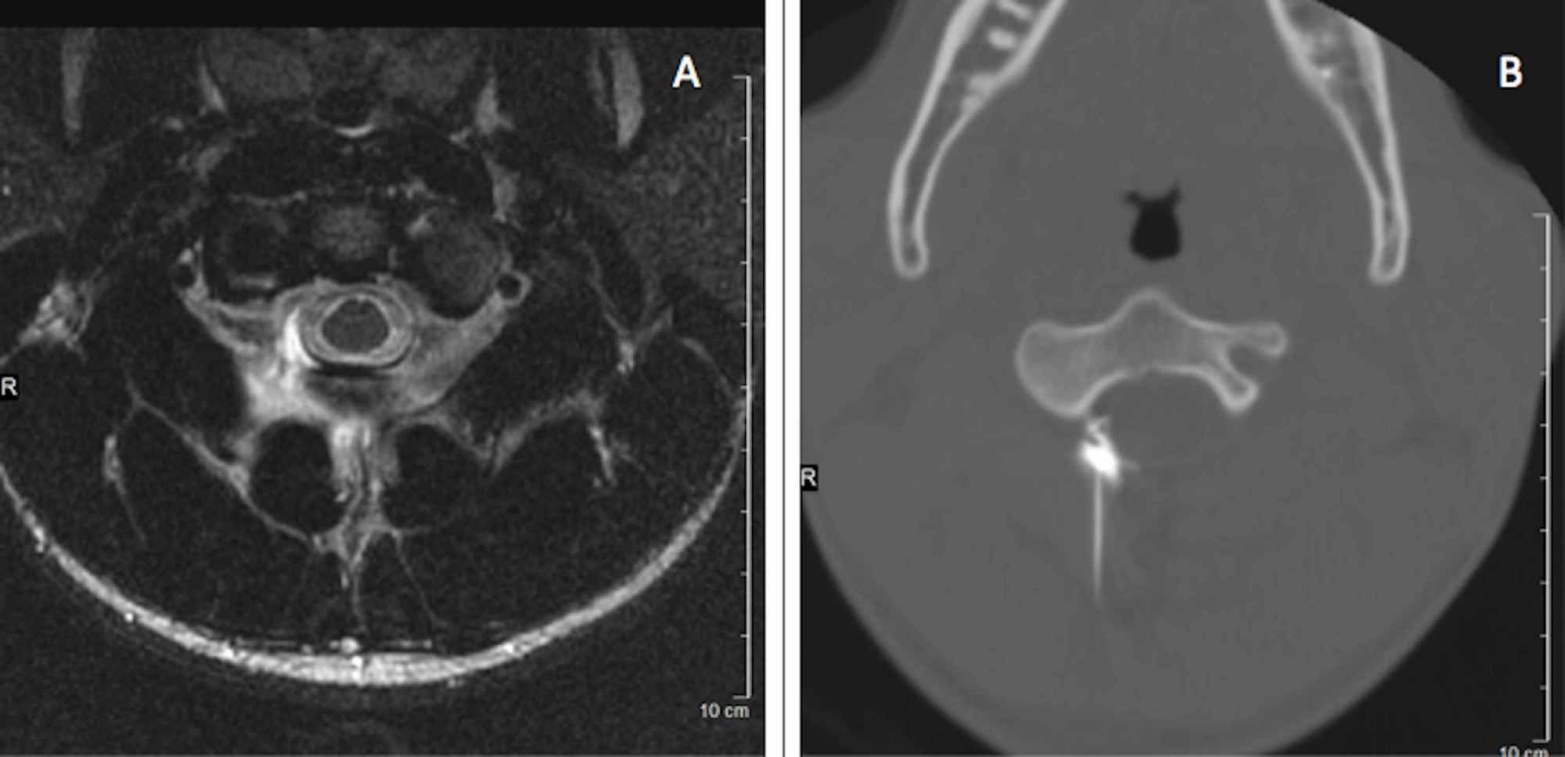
Magnetic resonance (MR) myelogram and CT-guided blood patch (A) T2-weighted MR myelogram image revealing a cervical cerebrospinal fluid leak. (B) CT-guided cervical blood patch targeted at the area of leak seen on MR myelogram.
